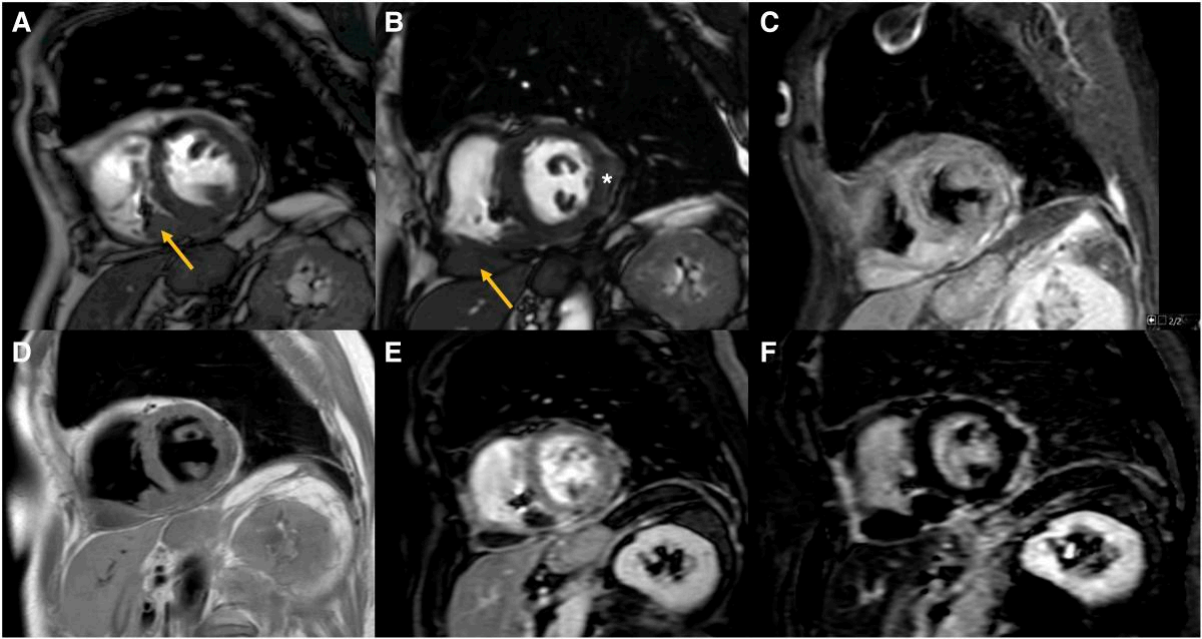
Cardiac mass imaging by cardiac magnetic resonance demonstrated across multiple sequences. (*A* and *B*) Short-axis cine images (balanced Steady-State Free Precession) showing a large mass involving the diaphragmatic wall of the right ventricle (yellow arrow), along with a smaller mass located in the mid-lateral wall of the left ventricle (asterisk). (*C*) T2-weighted sequence showing hyperintensity of the masses. (*D*) T1-weighted sequence showing isointensity of the masses relative to myocardium. (*E*) Early gadolinium enhancement images showing no contrast uptake. (*F*) Late gadolinium enhancement images showing absence of enhancement.
